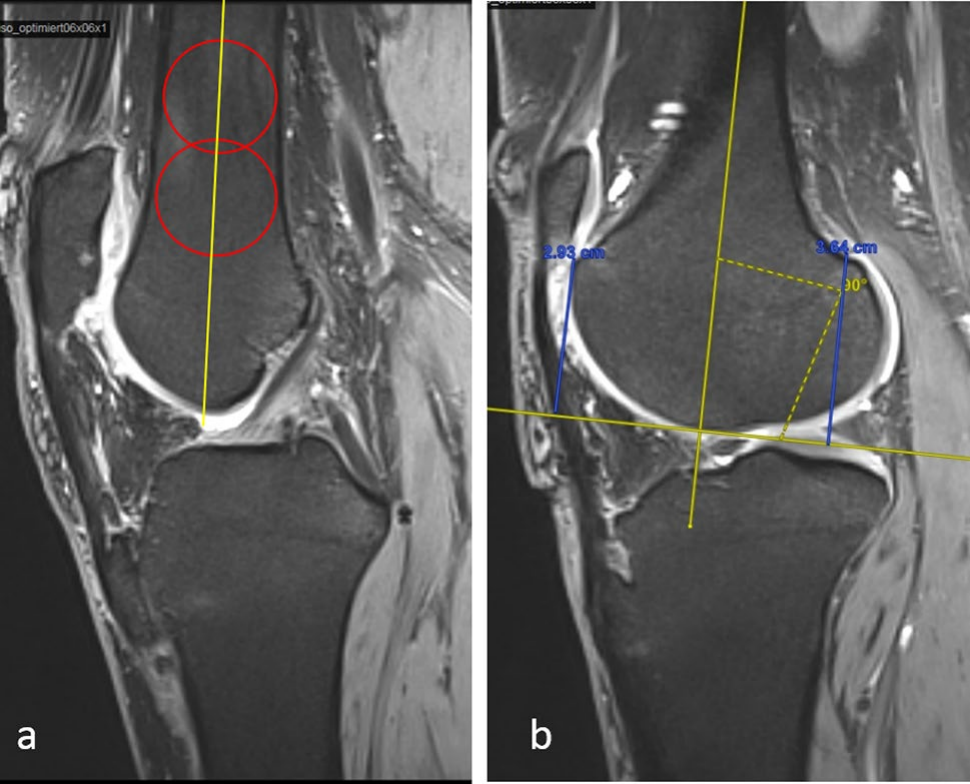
The lateral condylar index: two circles are drawn in a sagittal MRI image at the level where the anterior cruciate ligament is present at its entire length. The line connecting the centers of the two circles defines the femoral axis. A line vertical to the femoral axis tangential to the distal femoral cartilage serves as baseline to measure the length of the anterior (*a*) and posterior (*p*) cartilage length. The value (*a*/*p* × 100%) is the lateral condylar index. In this patient a pathologic lateral condylar index of 80% was calculated
